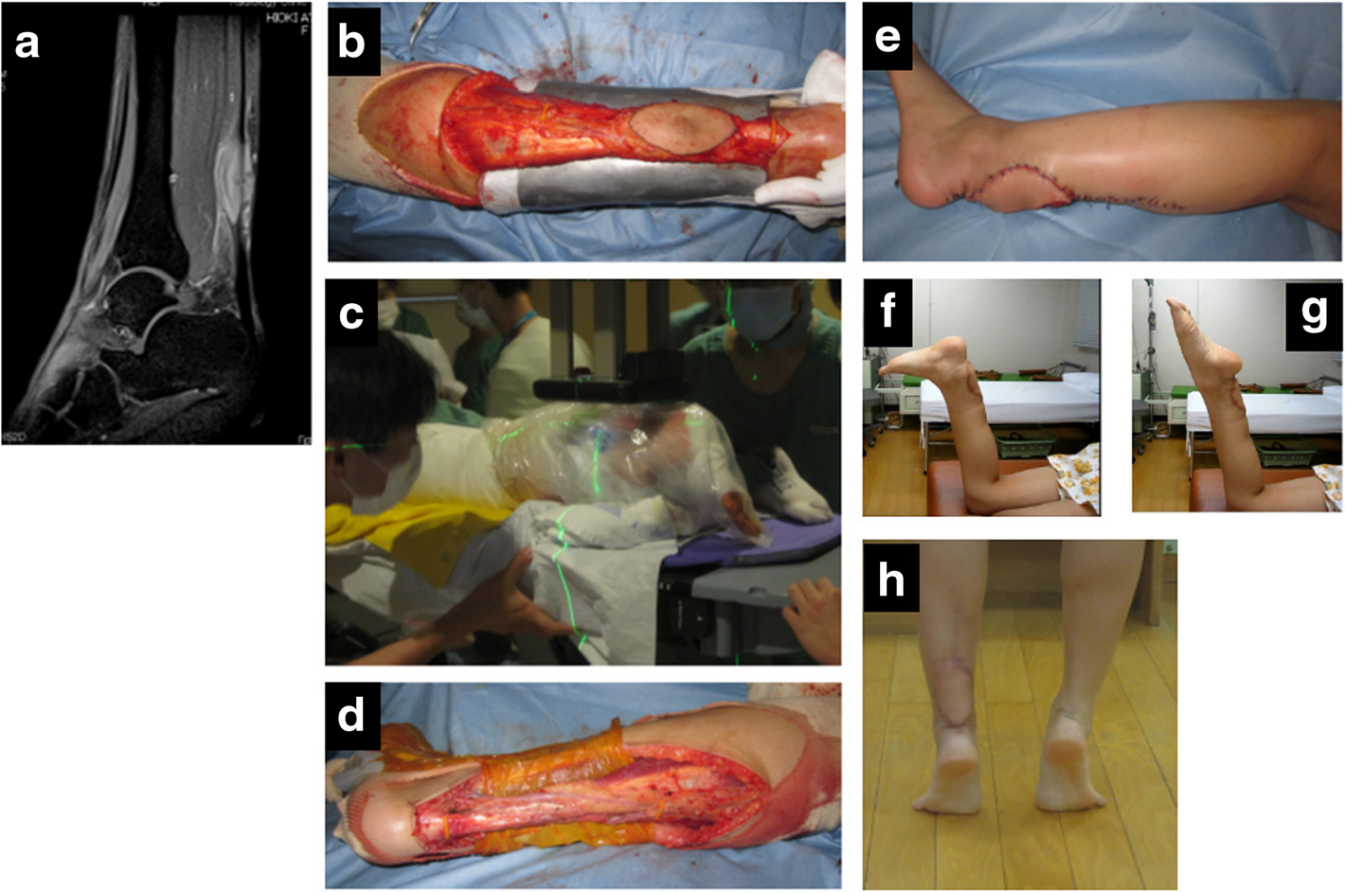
Surgical procedure and post-operative limb function in case 2, (a) T2 weighted-MR image showing clear cell sarcoma located intra-tendinously at calcaneal tendon. b After the elevation of the tumor mass, including the tendon and nerve from the tumor bed, a 6-mm-thick lead boards were inserted beneath the tumor mass to shield the normal tissue (c). The patients were transferred to the radiotherapy department after the affected limbs were wrapped in sterilized plastic bags, and 35–50 Gy of 12 MeV electrons were delivered. d After irradiation, the patients were returned to the operation room, and underwent the marginal/intra-lesional resection of the tumor, preserving the tendon and nerve. e The soft tissue defect was then reconstructed using a free musculocutaneous flap. The photographs at the final follow-up time showing the normal ankle movement (f and g) and ability of stand on toe (h)
